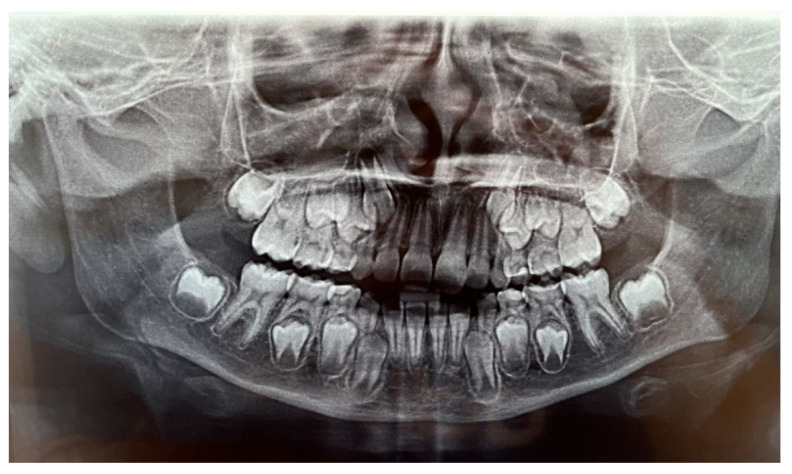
Orthopantomography X-ray before treatment (clinical case #4).
